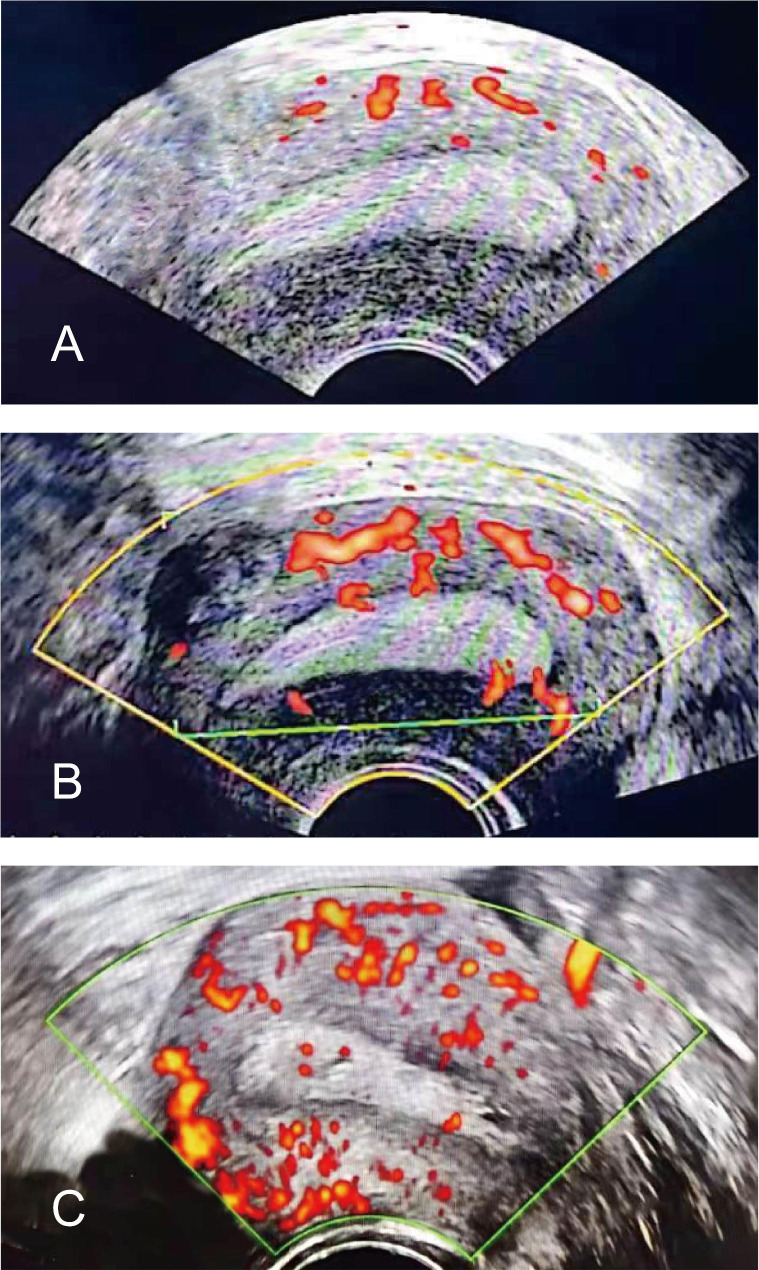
Power Doppler ultrasound imaging of endometrial and subendometrial blood flow: type-branch. (A) type I - branch<5: the vessels pass through the lateral hypo-echoic band of the endometrium but do not enter the hyper-echoic rim of the endometrium, branch 1. (B) type II - branch<5: the vessels pass through the hyper-echoic rim of the endometrium but do not enter the endometrium, branch 4. (C) type III – branch <5: the vessels enter the endometrium, branch 4
